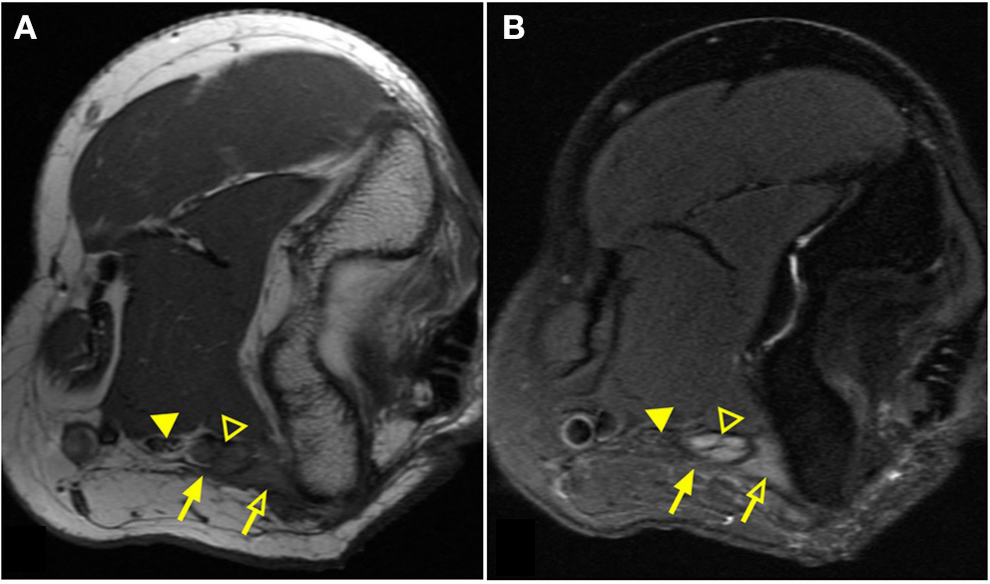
Magnetic resonance neurography (MRN) of the elbow demonstrates clear nerve abnormality. Elbow images of a patient with persistent/increasing ulnar nerve distribution symptoms after ulnar nerve transposition surgery. T1-weighted image (A) demonstrates scar tissue/fibrosis (solid arrow) around the ulnar nerve (open arrowhead) in greater detail. T2-weighted image (B) presents significantly increased signal of the ulnar nerve (open arrowhead) in comparison to the normal median nerve (solid arrowhead). Edematous muscle (open arrow) is clearly distinguished with increased signal on the T2-weighted image.
